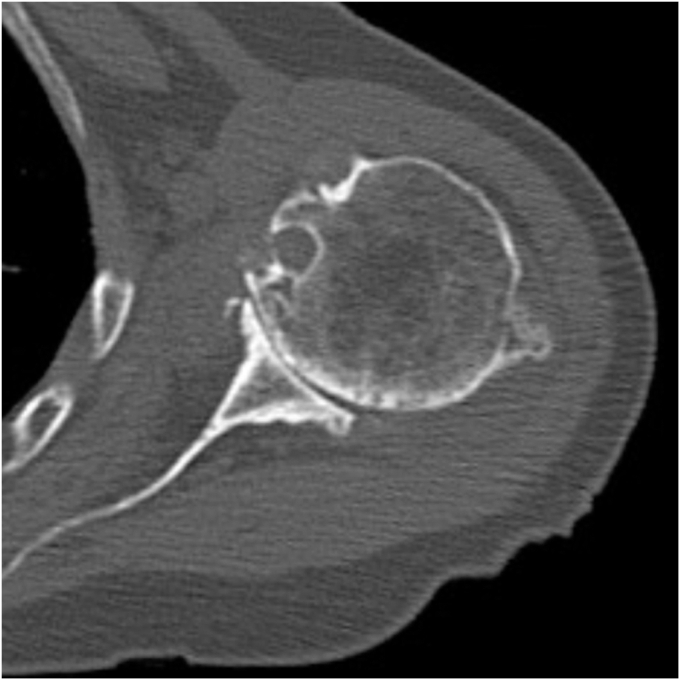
Preoperative computed tomography scans showed subchondral sclerosis of the glenohumeral joint and osteophytes around the bicipital groove.
